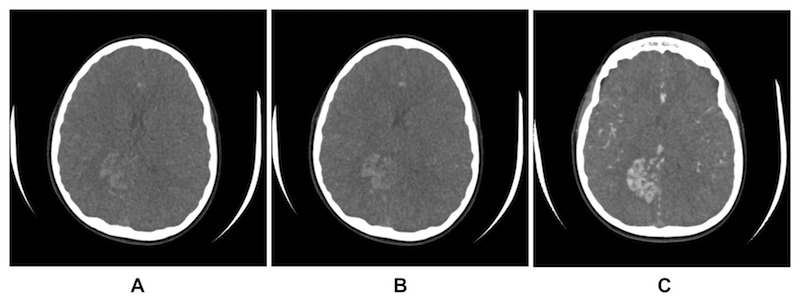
Time-resolved CTA (4D-CTA) for nidus identification. A representative case of treatment planning in an 11-year-old patient using pre-DOS 4D-CTA to identify the earliest filling phase (A) through last peak arterial phase and maximal intensity projection (MIP) images (B and C, respectively).
